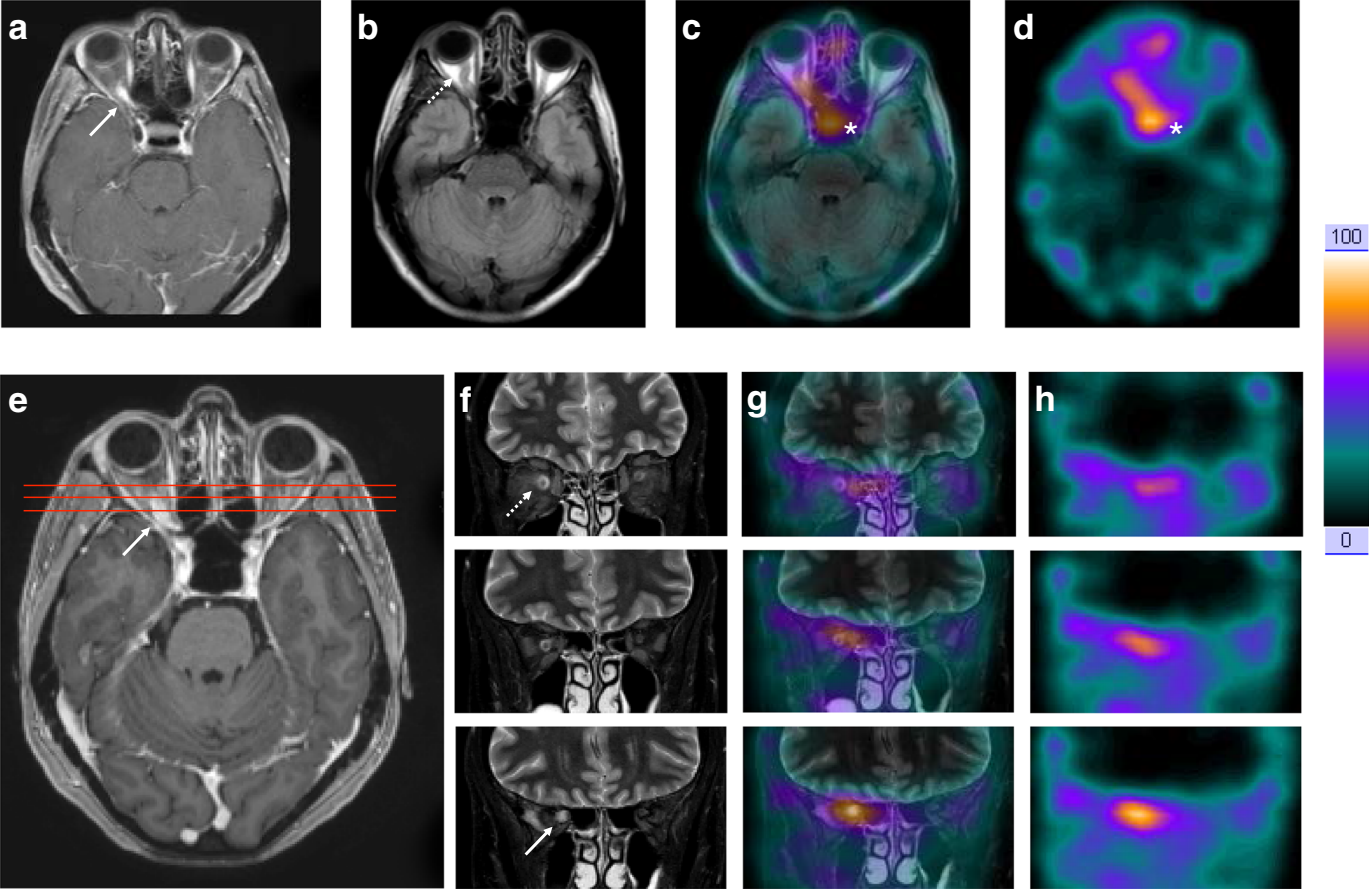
Multimodal imaging: matched transverse, postcontrast spin-echo T1-weighted (a), and fluid-attenuated inversion recovery (b) 1.5-T MRI scans, fused MRI/SPECT (c) and ^111^In-pentetreotide SPECT images (d). Transverse postcontrast gradient echo T1-weighted MRI scan (e) showing the location of coronal slices, and three-level coronal T2-weighted fat-suppressed MRI scan (f), fused MRI/SPECT image (g) and [^111^In]-pentetreotide SPECT image (h). MRI scan showed right tortuous optic nerve enlargement (b, *dotted arrow*), with a peripherally increased signal on fluid-attenuated inversion recovery (b, *dotted arrow*) and on coronal T2-weighted fat-suppressed sequences (f, *dotted arrow*). Therefore, uniform contrast enhancement (a and e, *solid arrows*) affected the last third of the nerve corresponding to an intense [^111^In]-pentetreotide uptake (d and h). The asterisk (*) shows the physiological uptake of [^111^In]-pentetreotide in the pituitary (c and d)
